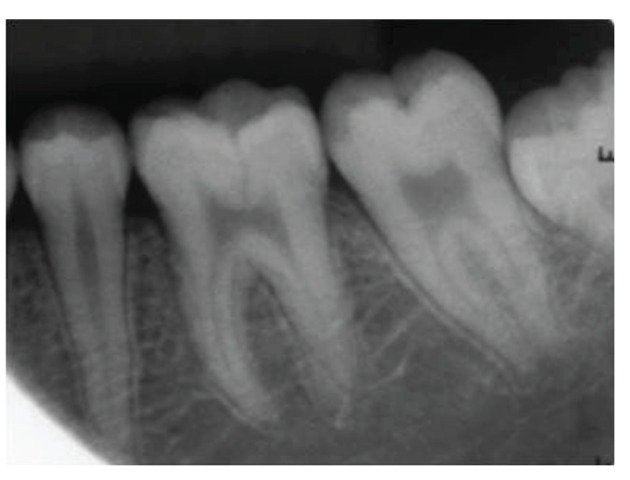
Preoperative periapical radiograph with 36 showing the radiolucent fracture line in the coronal area and passing through the pulp chamber.
